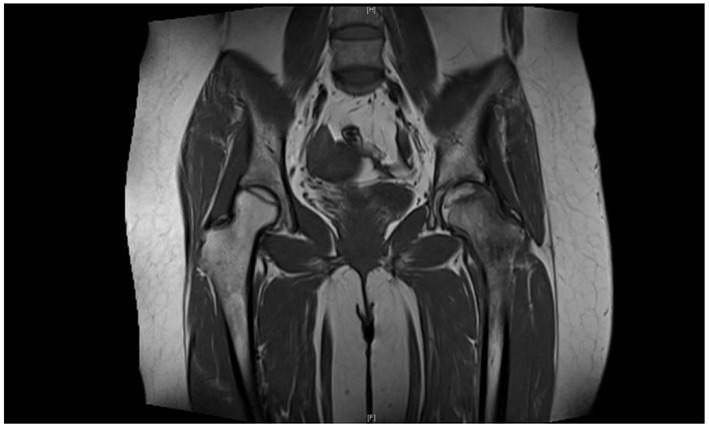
Bilateral hip MRI show bilateral ONFH.
